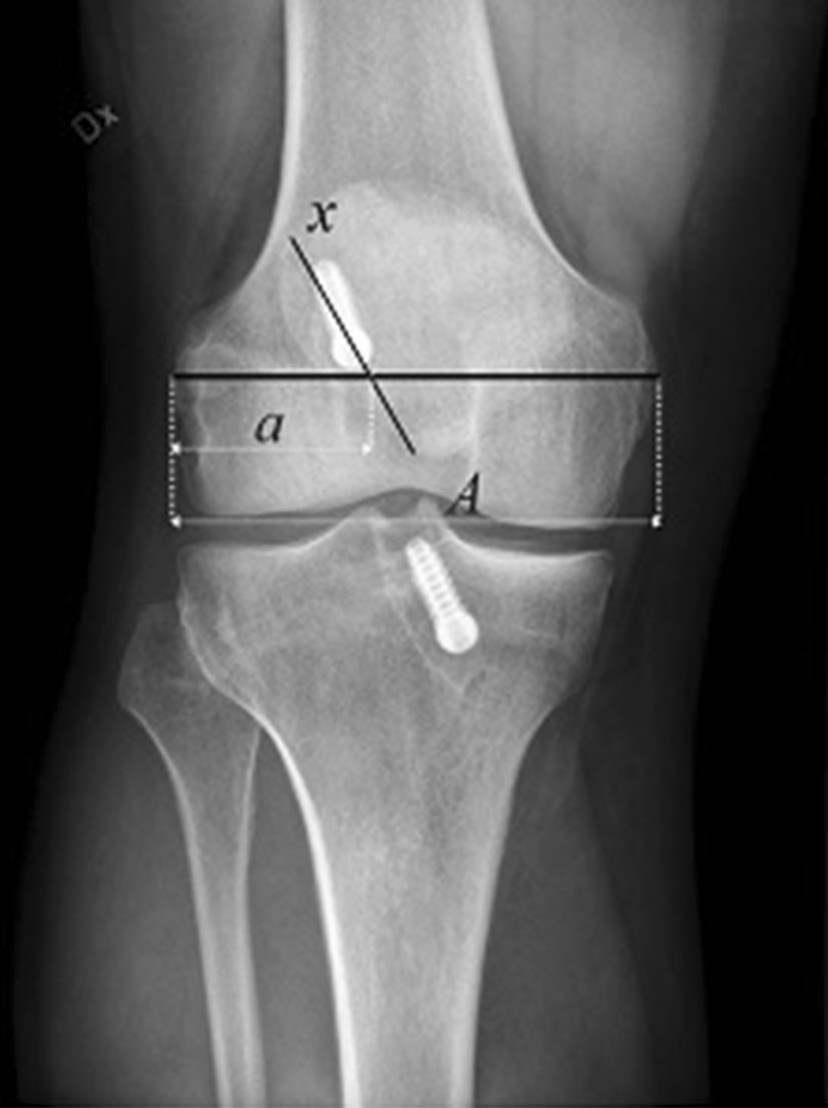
A radiograph showing the measurement technique for the femoral tunnel position in the coronal plane. The largest distance between the medial and lateral femoral condyles was identified (A). The distance from the lateral condyle to the center of the tunnel was measured (a). The obtained distance (a) was divided by the total width (A) and the position of the femoral tunnel was expressed as a percentage
